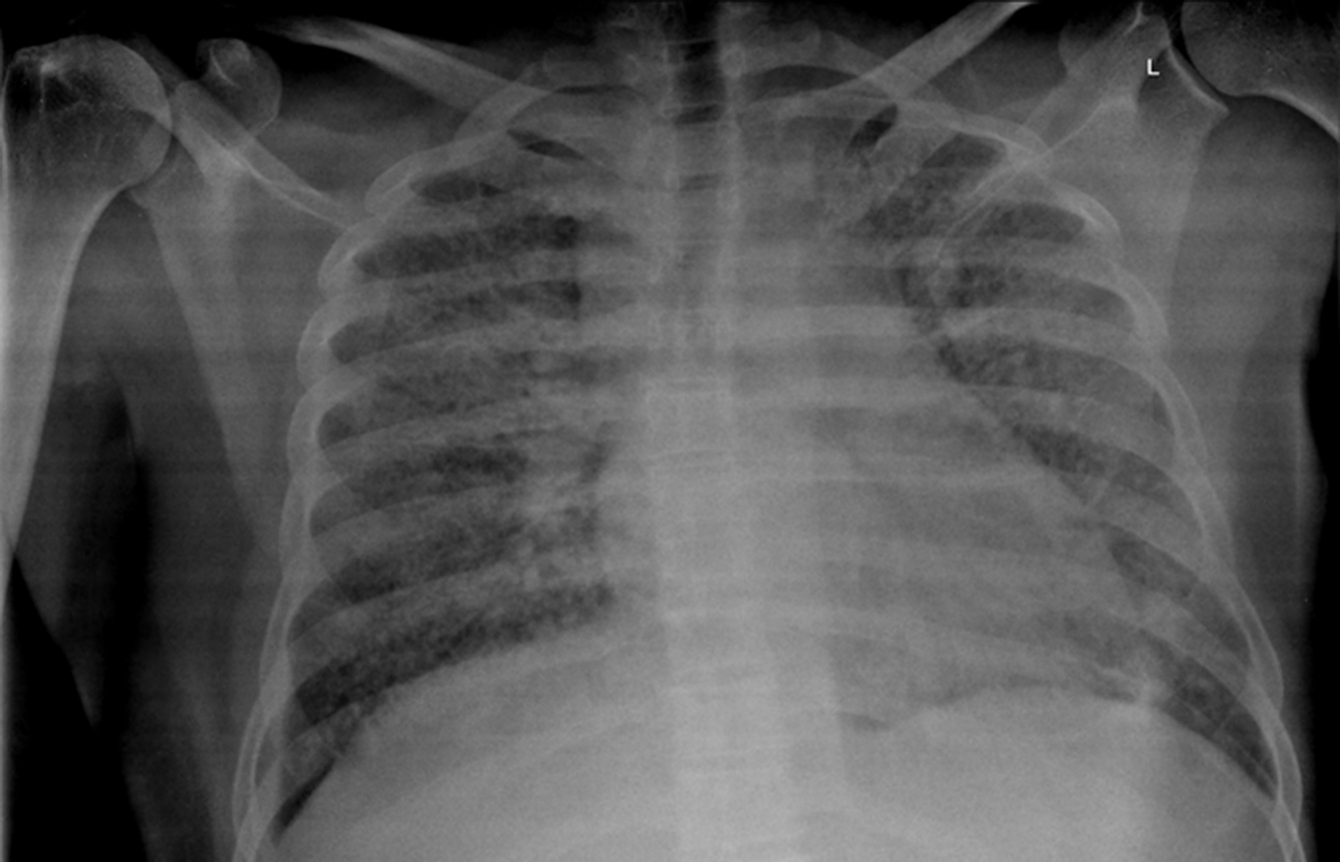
Case 1(a): Frontal chest radiograph showing multiple reticulonodular opacities in bilateral lung fields.
